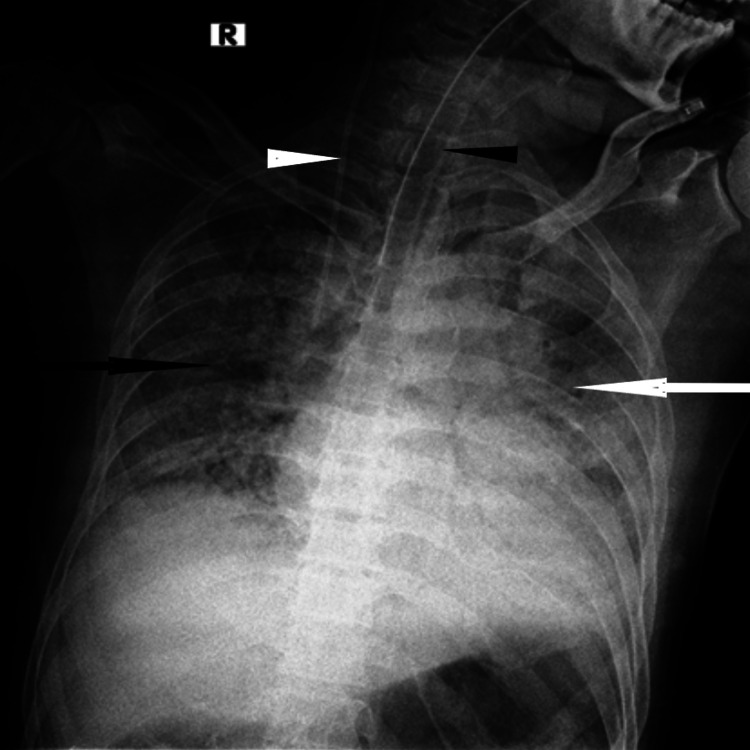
Case 3 Seventy-five years old COVID-19-positive patient had a history of fever and dyspnoea for 10 days. The patient underwent invasive ventilation. The white arrow shows diffuse consolidatory changes involving left lung parenchyma. The black arrow points to ground glass opacities involving the right lung parenchyma. Chest X-ray findings were suggestive of extensive pneumonia. The central line pointed by the white arrowhead and the endotracheal tube pointed by the black arrowhead can be seen.
